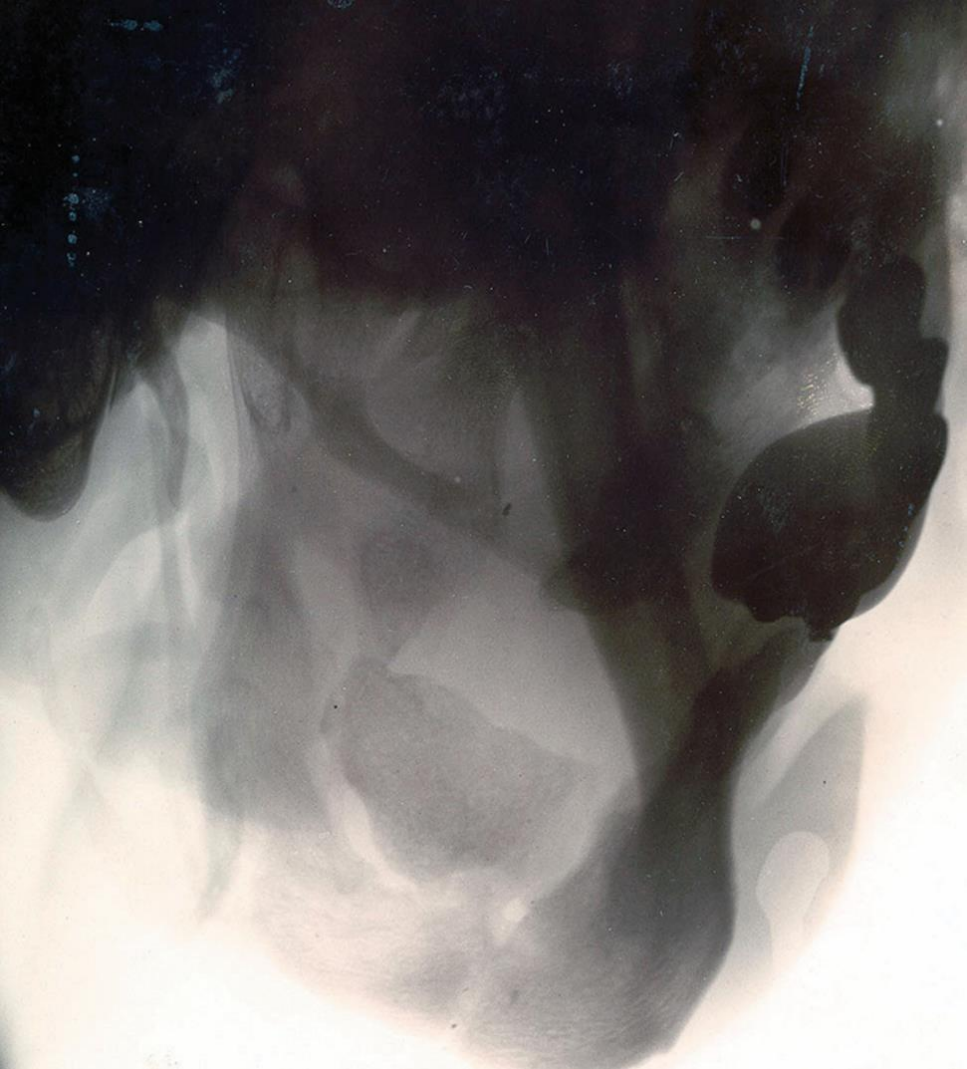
Plain radiograph demonstrating the bony injuries sustained by Gillies’ 23-year-old soldier.
